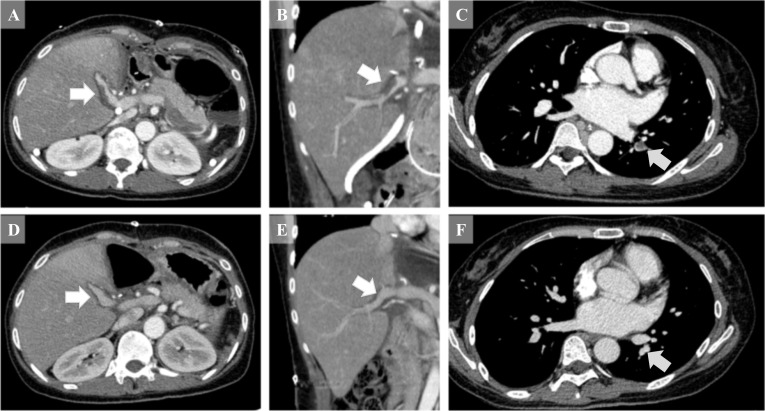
Partial thrombus in the portal (PV) and left renal veins revealed by computed tomography (CT). a–c Contrast-enhanced CT scans performed on postoperative day 5 revealed partial thrombus in the PV (white arrows) and the left pulmonary artery (grey arrow). d–f Contrast-enhanced CT revealed the disappearance of the thrombus in the PV (white arrows) and the left pulmonary artery (grey arrow) after 3 months
